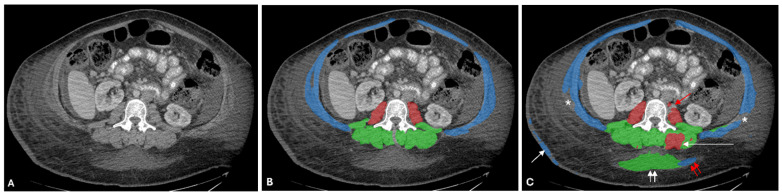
Algorithmic misclassification associated with subcutaneous edema. Representative axial L3 CT slice from the internal training dataset demonstrating localized boundary failures in a patient with altered soft tissue density. Panels display the unannotated CT (A), manual reference standard (B), and automated prediction (C). Target classes are color-coded (red = psoas, green = paraspinal, blue = abdominal wall). Spatial overlap was reduced across all classes, psoas (DSC: 0.655), paraspinal (DSC: 0.648), and abdominal wall (DSC: 0.744). The psoas prediction undersegments the left psoas (single red arrow) and bridges into the left paraspinal compartment (single long white arrow). The paraspinal boundary extends into the posterior subcutaneous edema (double short white arrows). The abdominal wall boundary undersegments the bilateral oblique muscles (*) and includes a focal region of left posterior edema (double short red arrows) and the right posterior skin (white arrow).
